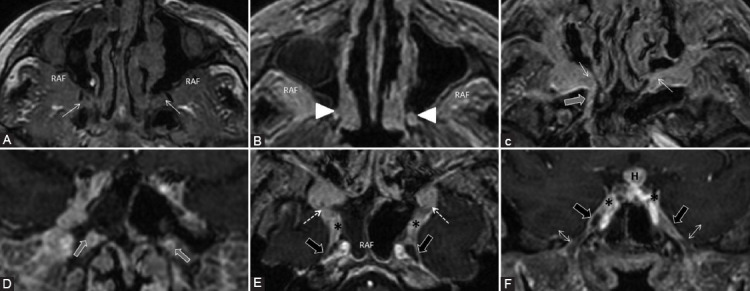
A 41-year-old male with IgG4-related disease with multiorgan manifestations: Central nervous system, orbital disease, sialadenitis, thoracic, and abdominal. Axial T1-weighted (A), axial (B, C, E), and coronal (D, F) contrast-enhanced 3D T1 images. Bilateral isointense infiltrative masses in the PPF (white arrows) and retroantral fat (RAF) are revealed, with corresponding homogeneous CE. There is enlargement and CE of great palatine (arrowheads) and vidian nerves (gray arrows). Enhancing tissue is observed also at the cavernous sinuses (*) extending to the rotundum foramen (dashed arrows), while Meckel’s cave appears normal (black arrows). The foramen ovale is also spared and normal hypointense CN V3 can be noticed surrounded by the contrast filled venous plexus (double arrow). Symmetric pachymeningeal thickening and enhancement are present. Notice the enhancement of the hypophysis (H).
